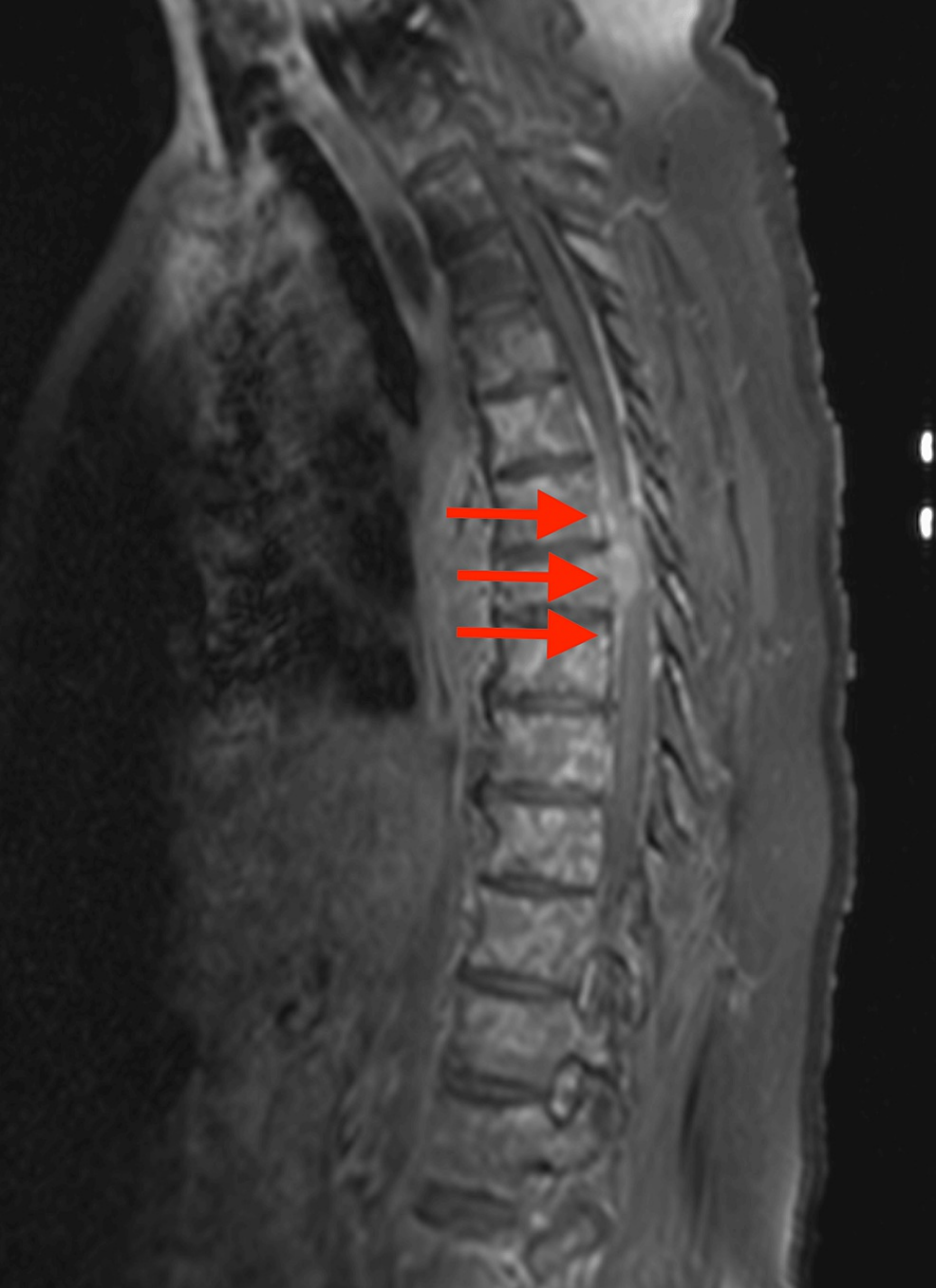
T1-weighted sagittal MRI with IV contrast showing evidence of erosion of neoplasm through the posterior endplate into the adjacent anterior epidural space, where there is associated enhancing extramedullary neoplasm with associated spinal canal narrowing
